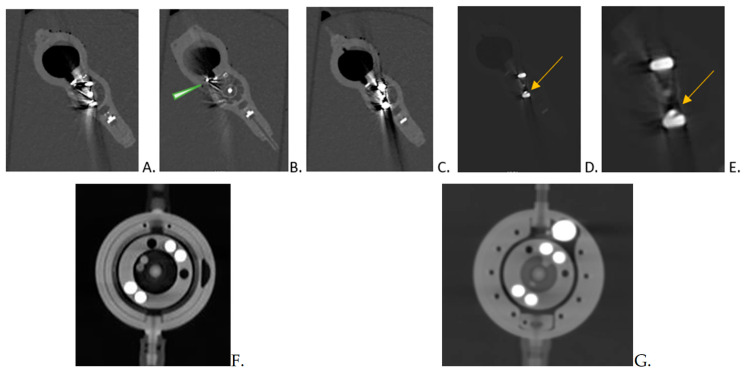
Ex vivo imaging to demonstrate the imaging possibilities of photon counting CT: Codman Certas (A–E) with proGAV (F) and M.blue (G) Codman Certas imPC CT imaging using slices of 0.5 mm thickness. A special emphasis is placed on the magnet marking, indicated by a tantalum ball serving as a setting indicator (arrow) in images (C,E), and a marker on the right-hand side (arrowhead) in image (B). It is important that the settings depicted in images (D,E) are consistent with those in the other pictures of the valve (A–C). Only using the unique capabilities of photon counting, which offer enhanced brightness and adjustments in grayscale, was it possible to accurately interpret the readings.
